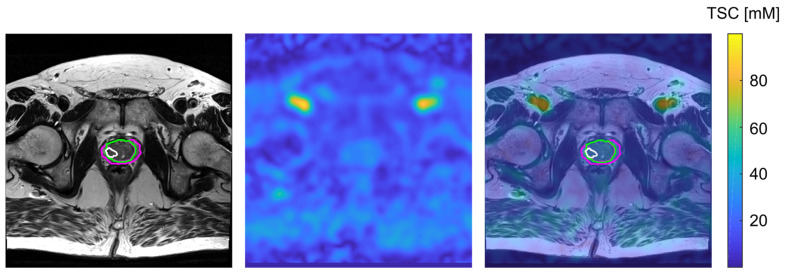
Transverse slice of the T2w TSE MR image of one patient with the segmentation of PZ (pink), TZ (green), and one lesion (white) within the TZ (left), the corresponding co-registered and quantified ^23^Na MR image (middle) and the overlay of both images, including the segmented regions (right).
